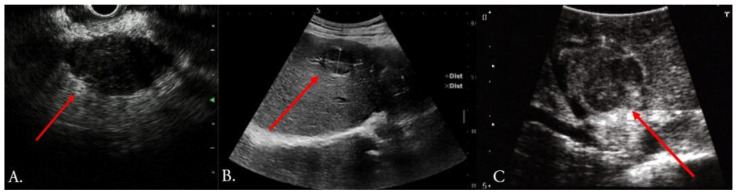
Commonly used ultrasound methods (red arrow indicates mass): (A) endoscopic ultrasound of pancreatic lesion, (B) preoperative transabdominal ultrasound of HCC liver lesion, (C) intraoperative ultrasound of HCC liver lesion close to the vasculature (identical lesion to image (B)). Original figure.
